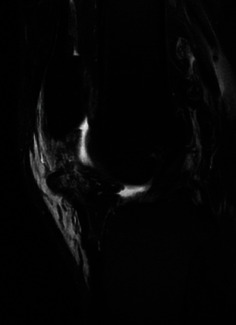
Fat-suppressed T2-weighted sagittal magnetic resonance image of the left knee shows rupture of the left patellar tendon.
